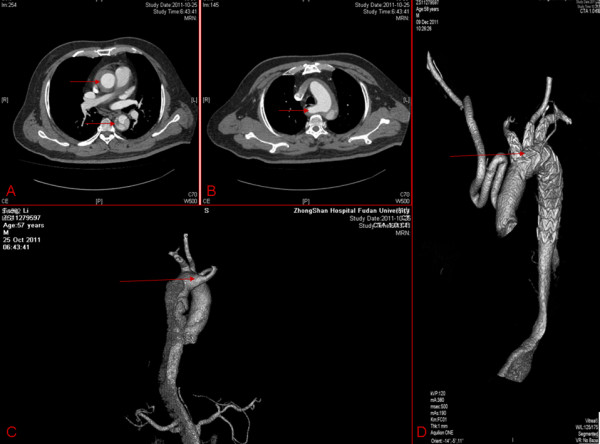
The patient's CTA before and after the operation. A: Standford type A aortic dissection; B, C: aberrant right subclavian artery; D: good position of triple-branched stent graft after operation.
